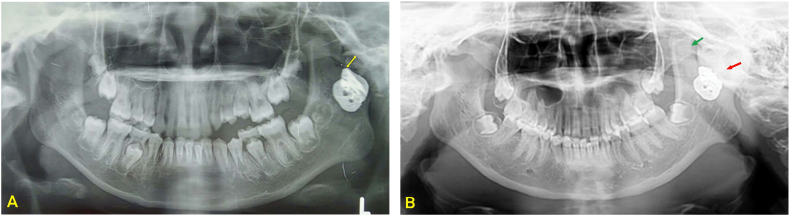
A Panoramic radiograph was taken immediately after the first arthroplasty. There was a radiopaque image of metal on the left mandibular ramus showing a gold foil as interposition material and there was a clear gap between the mandibular fossa and the ramus (yellow arrow), B Preoperative panoramic radiograph was taken 5 years after the first surgery. The gap between the mandibular fossa and ramus was not visible, filled by bone that extends over the mandibular incisura (red arrow). Hyperplasia or elongation of the left coronoid processus is visible (green arrow). (For interpretation of the references to colour in this figure legend, the reader is referred to the web version of this article.)
